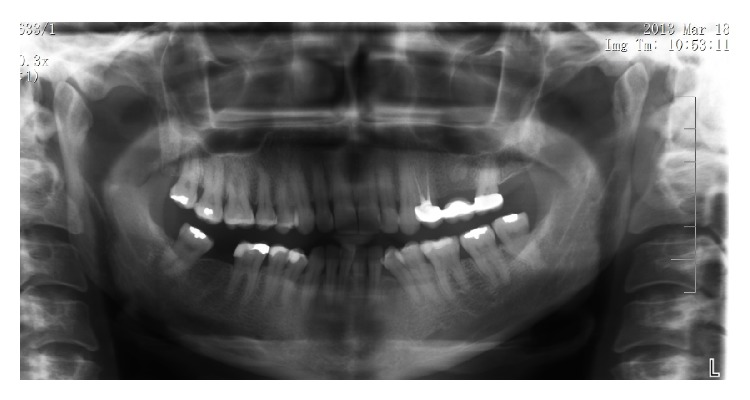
Panoramic radiography showed evidence of a previous root canal therapy with periapical radiolucency.
